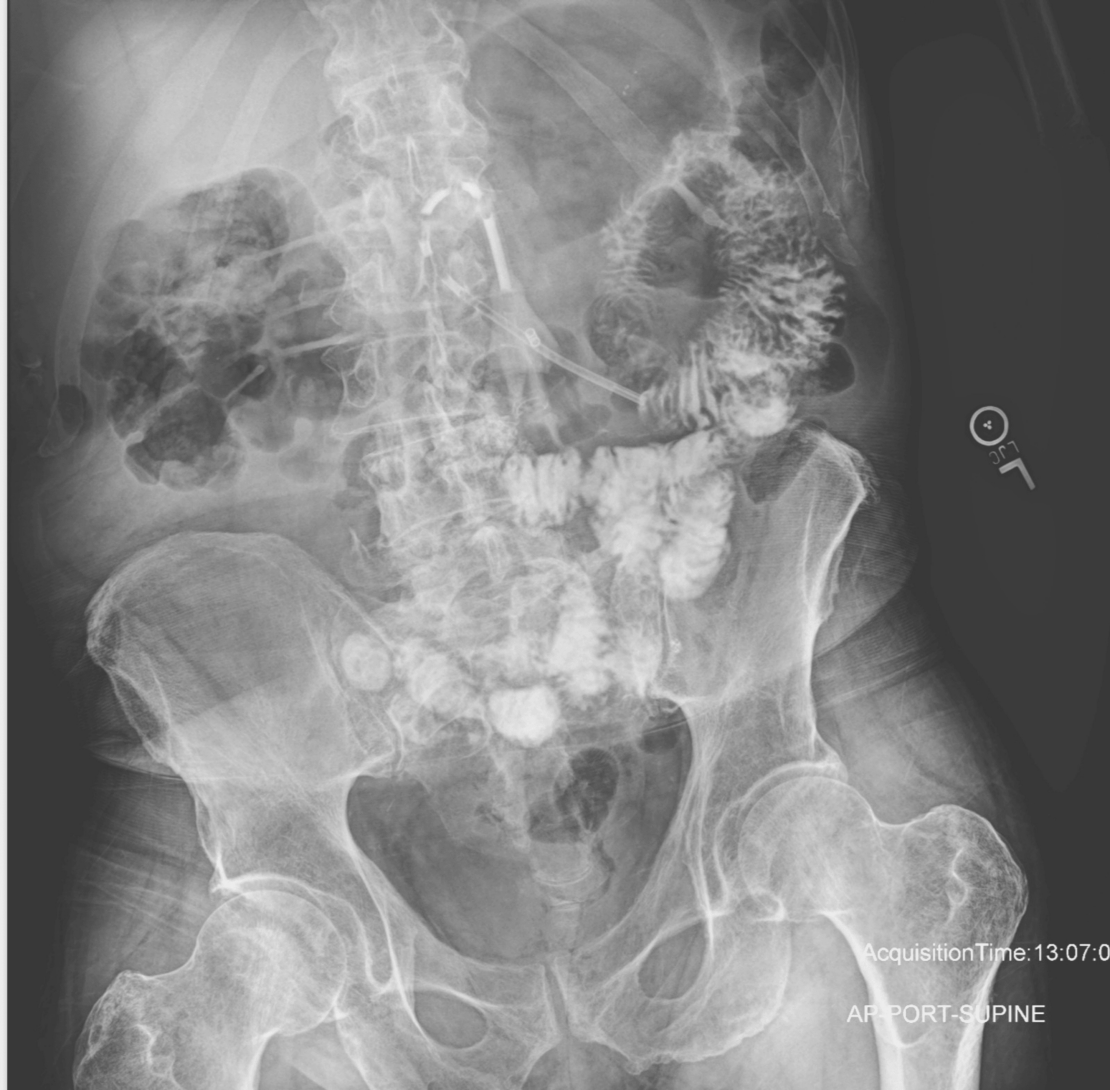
Abdomen and pelvis anterior-posterior single portable X-ray view Single portable AP, passive view of the abdomen and pelvis obtained after injection of contrast through the feeding tube, showing contrast within the PEG tube and multiple non-dilated small bowel loops over the left upper quadrant and mid-abdomen. No contrast was seen in the stomach or distal small bowel, and no extra luminal contrast was identified. PEG: Percutaneous endoscopic gastrostomy
